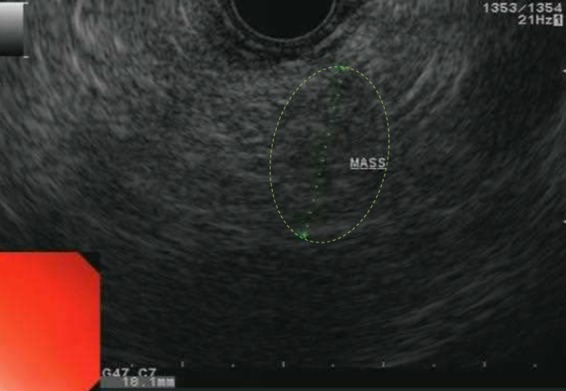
Endoscopic ultrasound. Isoechoic, heterogeneous, mixed solid, and cystic mass in the neck of the pancreas. Largest dimensions 23 mm by 21 mm.
